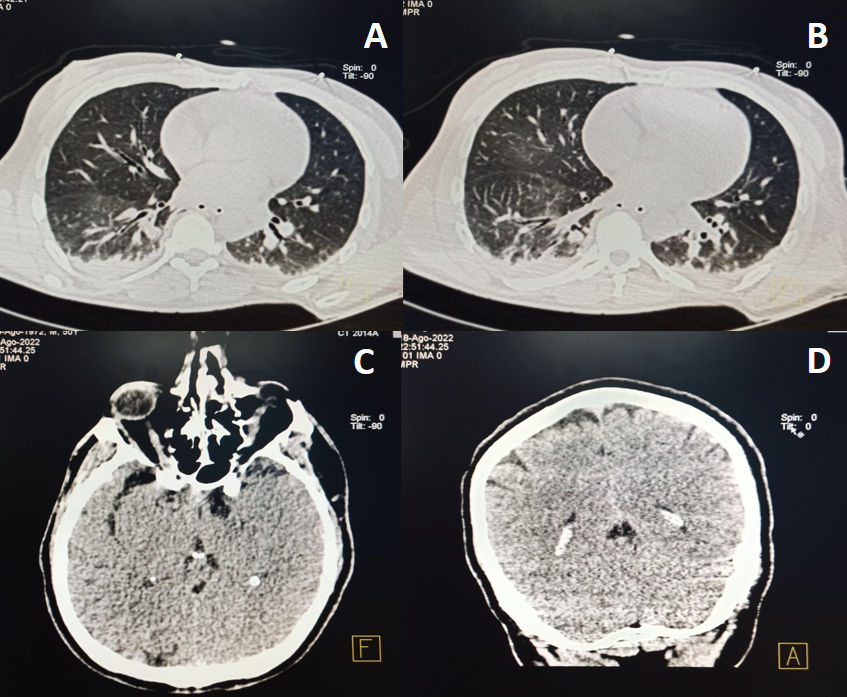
(A, B) Computed tomography (CT) of the chest with heterogeneous hypodense images with aerial bronchogram in both lungs more evident in the lower regions and related to an inflammatory process. (C, D) Cranial CT without cranio-encephalic lesions.
